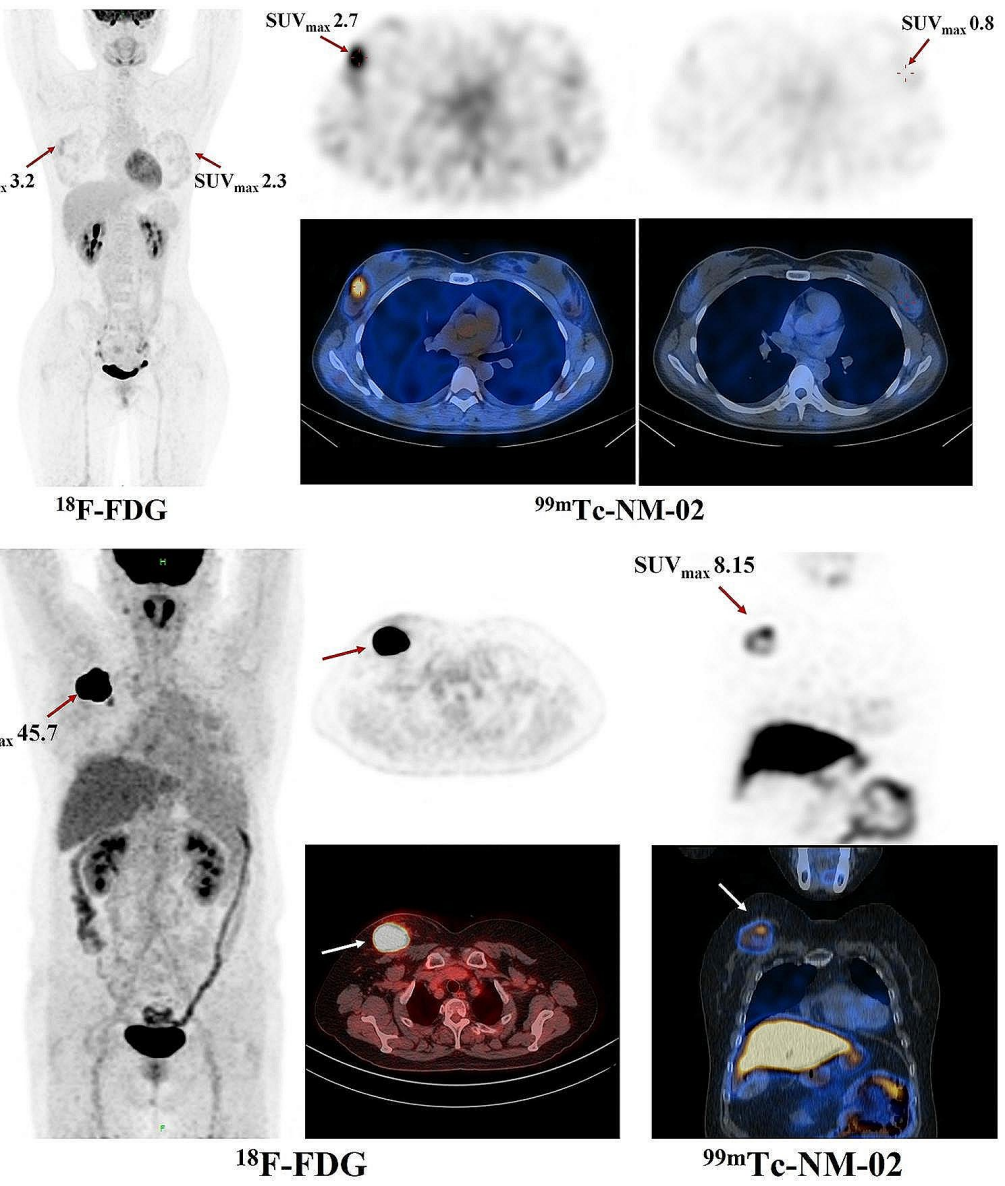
^18^F-FDG PET/CT and ^99m^Tc-NM-02 SPECT/CT of Patient BR014 and Patient BR037 at 1 h post-injection
